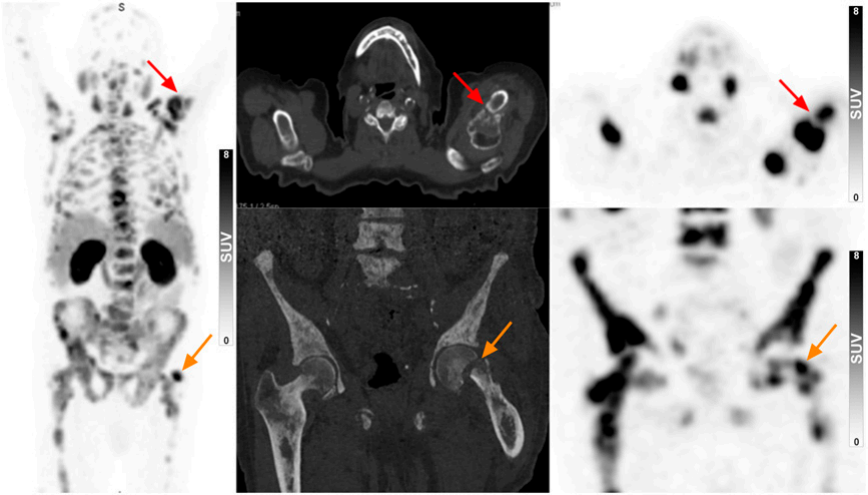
^68^Ga-PSMA-11 PET/CT of 82-y-old B(−) man with bone pain and PSA levels of 960 ng/mL. Not only effective in identifying disease extent, study also detected pathologic fractures of left humerus and left femur (arrows). Patient was immediately referred for radiotherapy for humeral lesion and surgery for femoral lesion.
